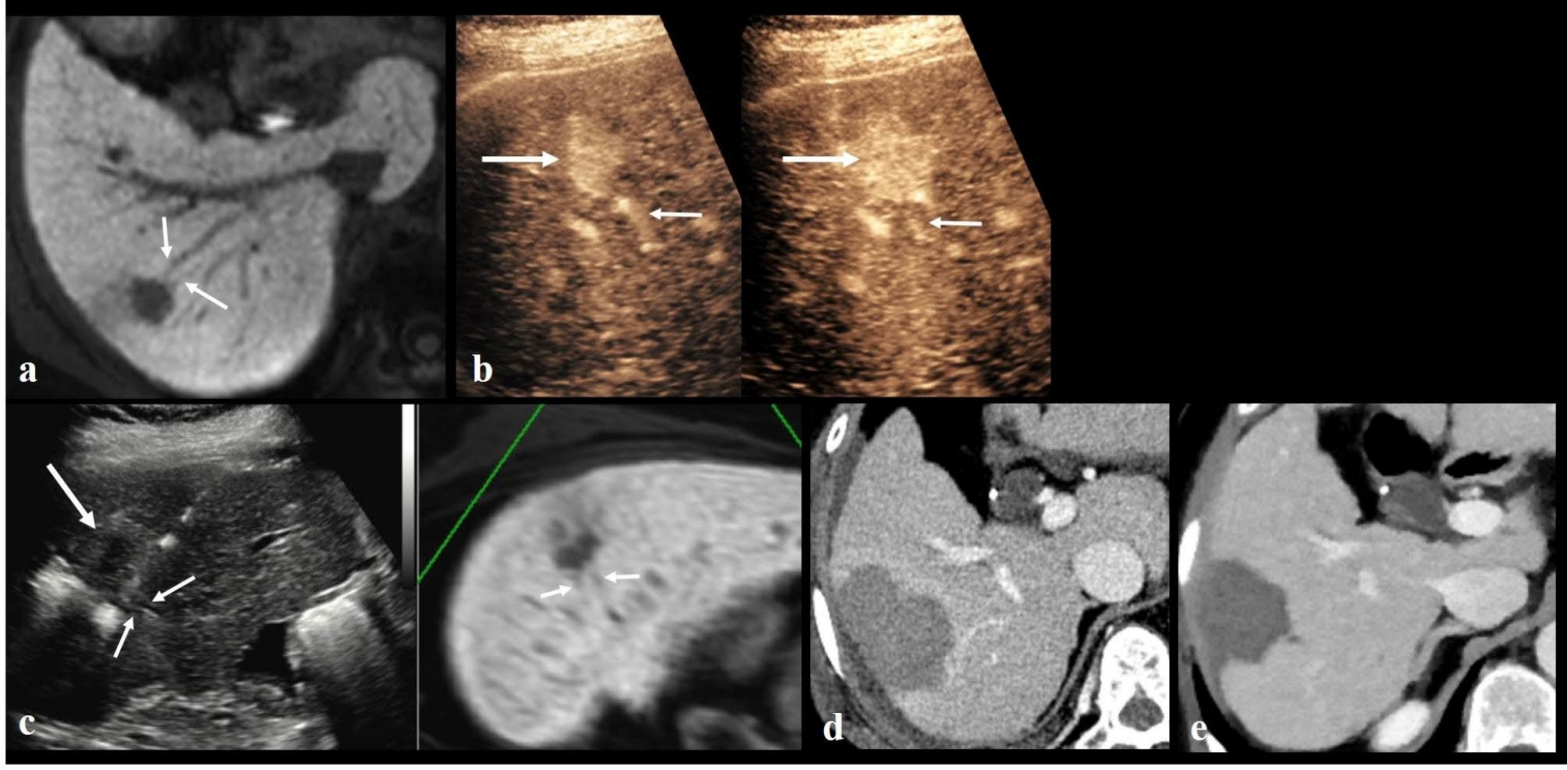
Anatomical radiofrequency ablation in a 70-year-old woman with a 2.0-cm hepatocellular carcinoma (HCC) and hepatitis B-related cirrhosis. (A) Hepatobiliary phase image of gadoxetic acid-enhanced MRI shows a 2.0-cm low signal intensity HCC in segment VI of the liver. Two tumor-bearing portal vein 4th branches are noted (arrows). (B) Contrast-enhanced ultrasound (Sonazoid) images well visualize the tumor (large arrows) and tumor-bearing portal vein branches (small arrows). (C) Real-time US-MR fusion image shows the low echoic target tumor (large arrow) and electrode placed across the tumor-bearing portal vein branches (small arrows). (D) Portal venous phase coronal image of immediate CT scan shows complete ablation of the target tumor and tumor-bearing portal vein branches with a sufficient safety margin (> 5 mm). (E) No local tumor progression was observed at 21-month follow-up CT.
